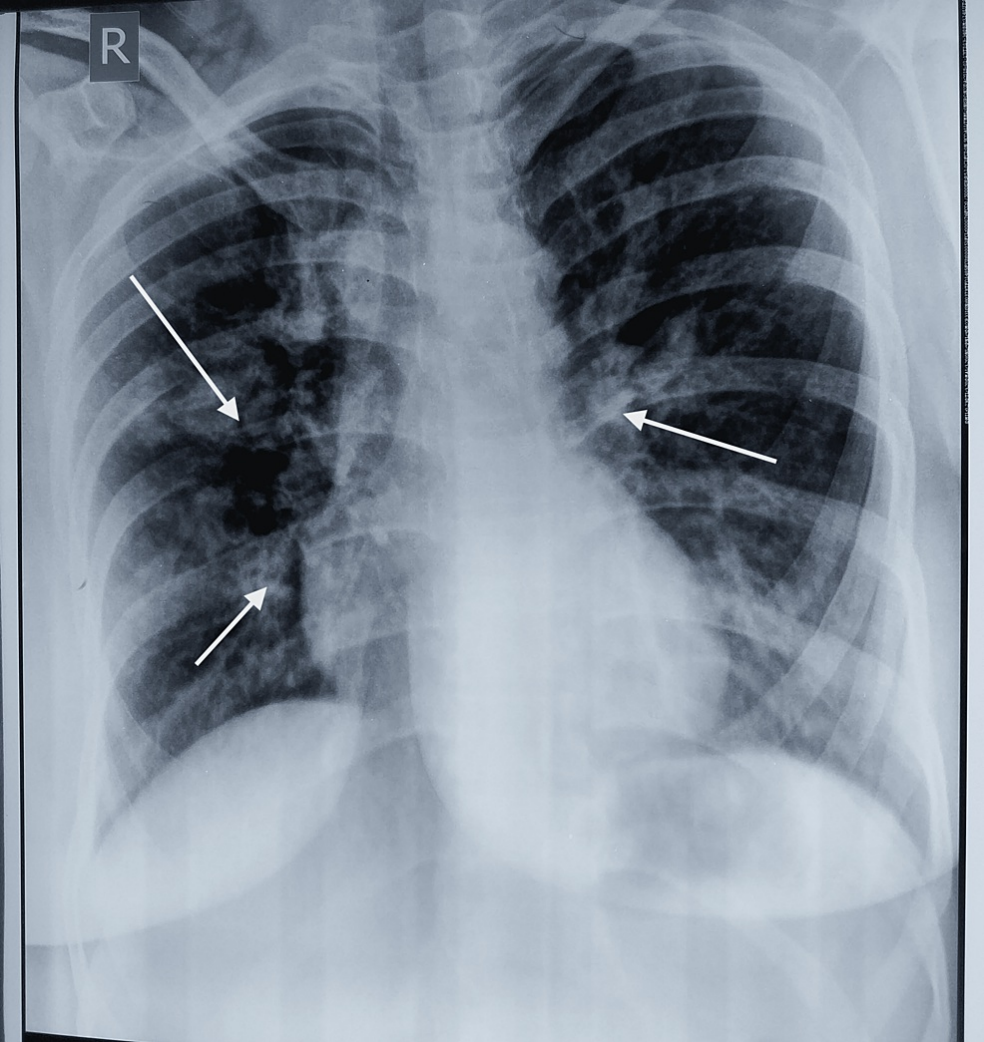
Chest X-ray of ABPA patient with central infiltrates and fleeting opacities (marked with white arrows). ABPA: Allergic bronchopulmonary aspergillosis
